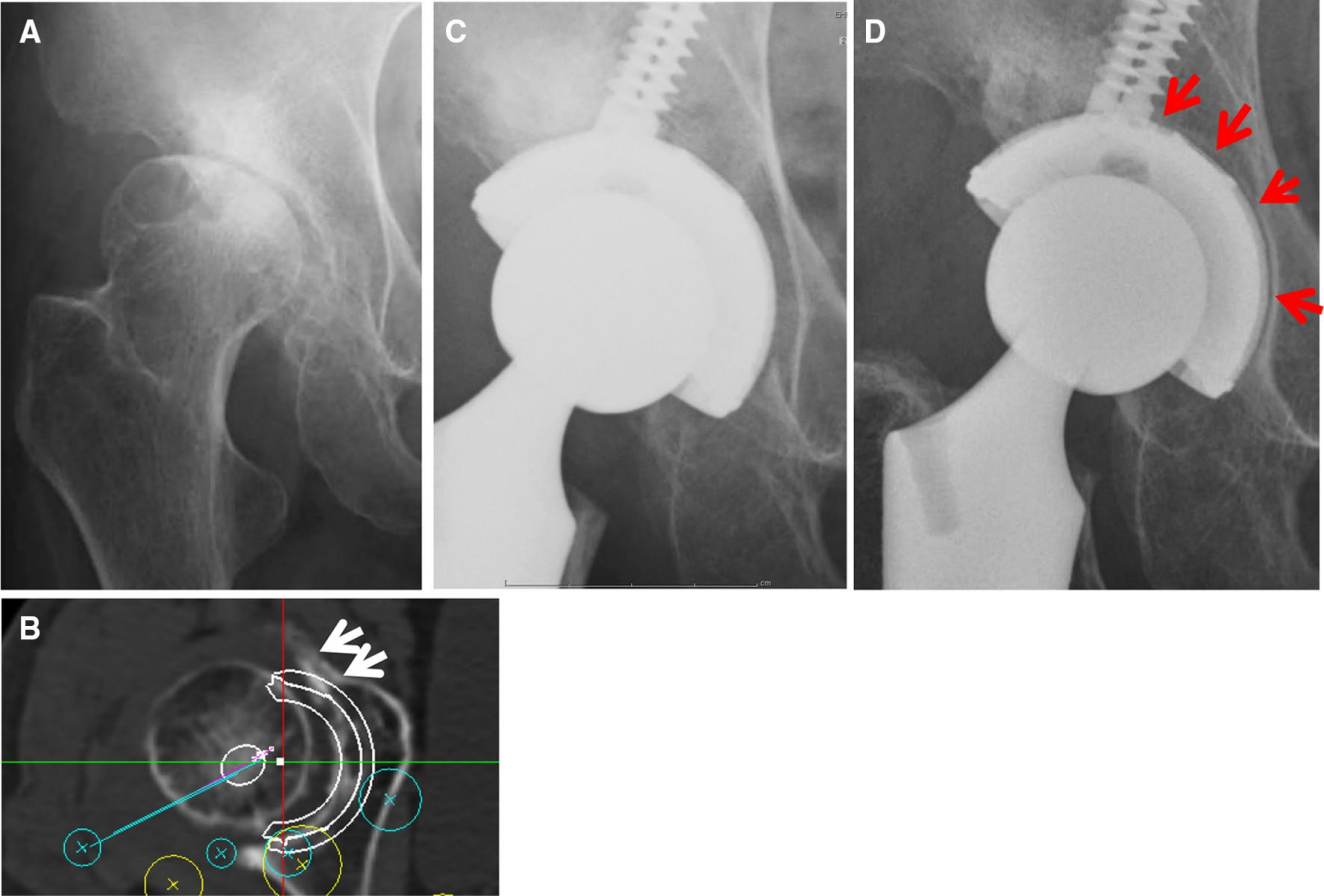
a A radiograph image of a 62-year-old-female patient with developmental dysplasia of the hip with atrophic bone remodeling pattern. Preoperative anteroposterior view. b The preoperative osteophyte of the anterior acetabular wall (white arrow). Preoperative CT image. c Postoperative anteroposterior view at 1-week follow-up. THA was used a highly porous socket with clustered screws (SQRUM TT™ socket; Kyocera Medical). There were no initial gaps between the acetabular socket and the acetabular host bed. d At 2-year follow-up, the radiolucent lines were seen in two DeLee and Charnley zones (red arrow)
